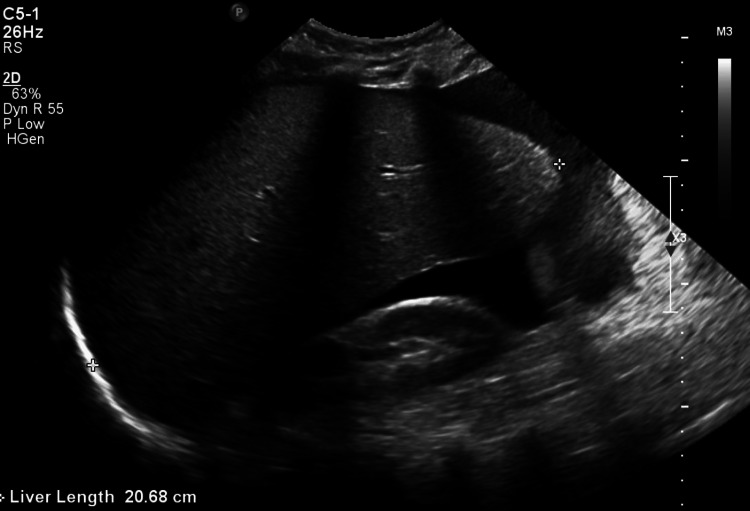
Abdominal ultrasound (US) findings: The visualized portions of the liver are homogeneous. There is hepatomegaly measuring 20.68cm and subtle hepatic surface nodularity suggestive of cirrhosis with a moderate volume of ascites (prior to conservative therapy).
